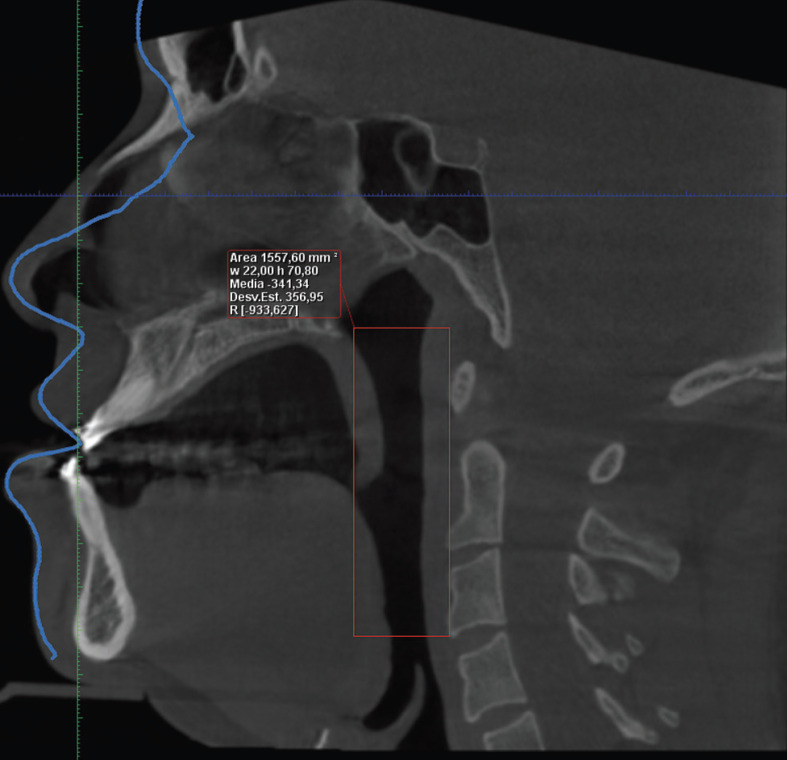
Referential image of CBCT in sagittal view with limits of the UA determined.
